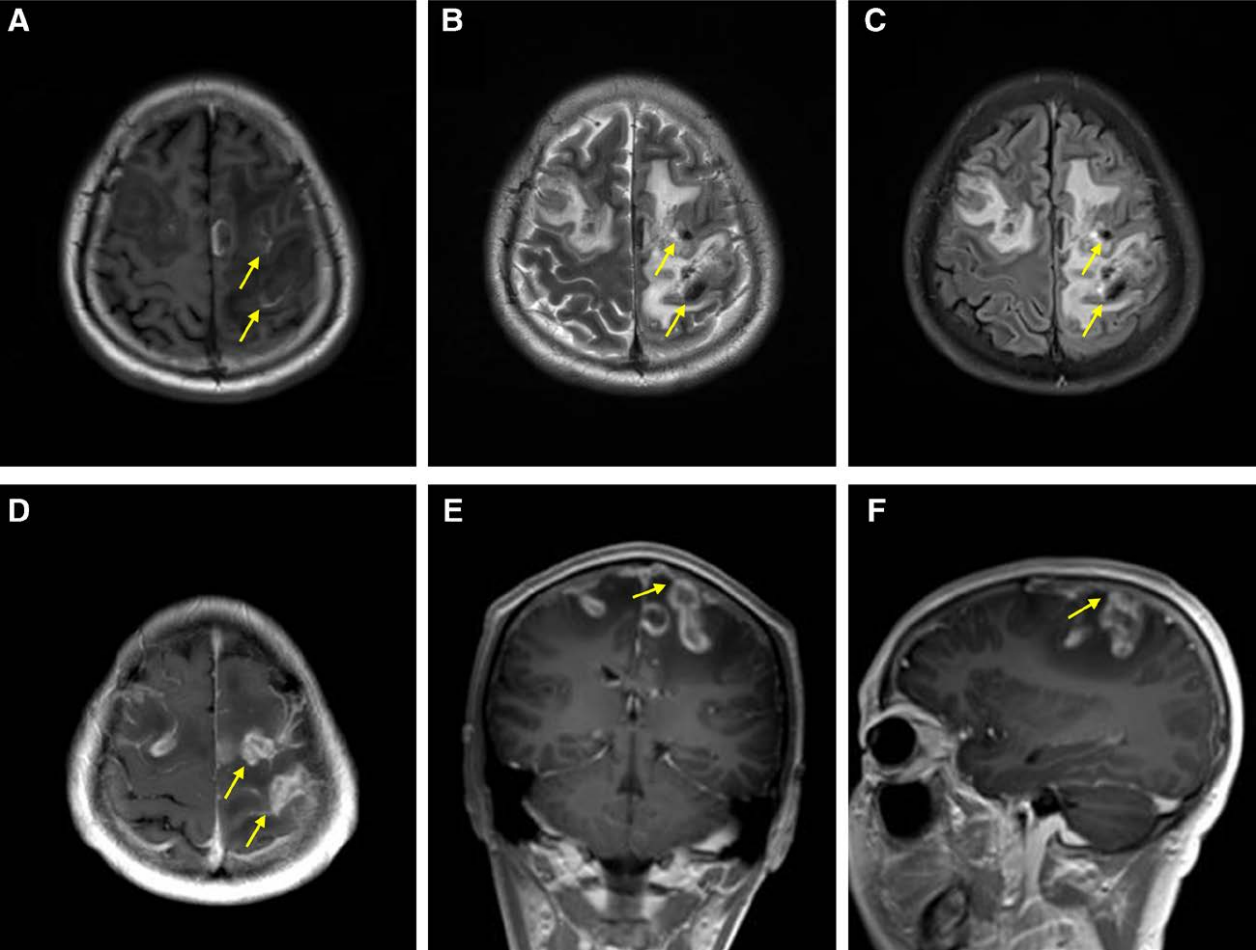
Images showed abscess was connected to the superior sagittal sinus. (A) MRI showed multiple ring-enhancing lesions on T1WI. (B) MRI showed multiple ring-hypointense lesions on T2WI. (C) MRI showed multiple ring-hypointense lesions on FLAIR. (D) Enhanced MRI showed multiple ring-enhancing lesions on T1WI. (E) Enhanced MRI showed abscess was connected to the superior sagittal sinus on T1WI coronary view. (F) Enhanced MRI showed abscess was connected to the superior sagittal sinus on T1WI sagittal view. MRI = magnetic resonance imaging, T1WI = T1-weighted imaging.
